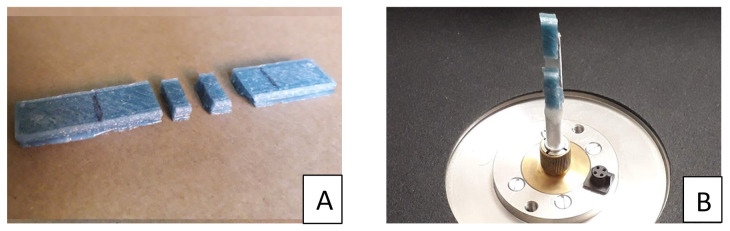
Cut samples for MICRO CT analysis (A) and housing in the tomograph holder (B).
